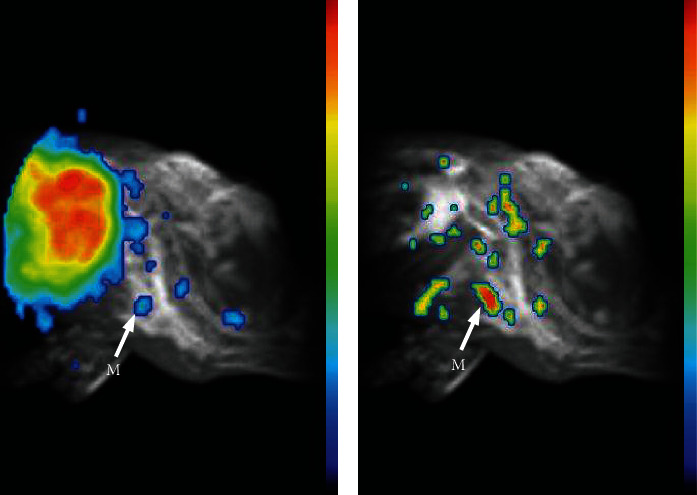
A MIP of the overlaid ^19^F signal over the anatomical scan in 1.5 Tesla MRI: (a) The fluorine in the liver is predominant on the image, and small signals in other organs are merely visible. Through cluster analysis, unspecific signals can be identified and subsequently excluded from the visualization by recomputing the colormap, leading to (b). The visual identification of weaker ^19^F enhancements (the marker M stands for the signal in the myocardium in this example) is thus facilitated.
